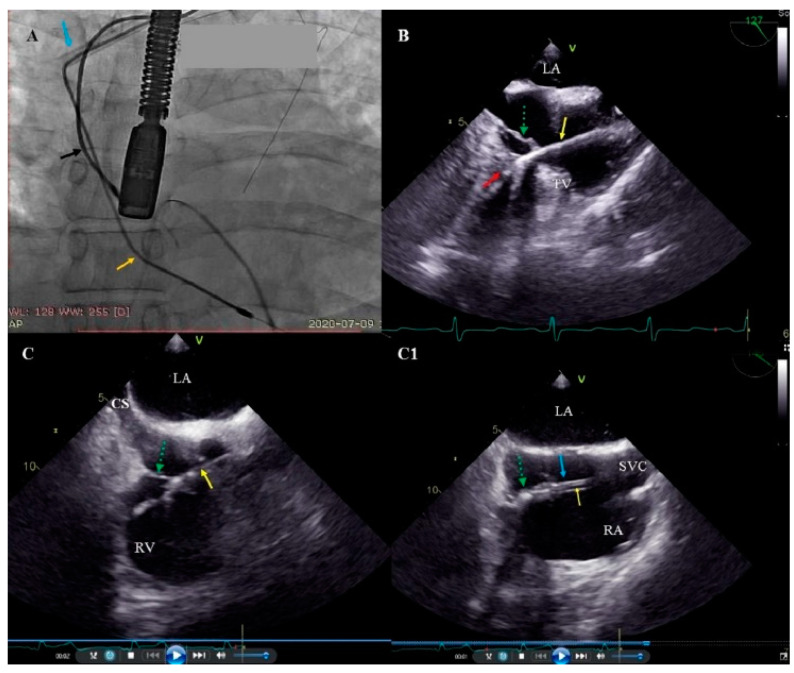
Pulling-up of the tricuspid valve (TV) leaflet while removing the lead adhered to the tricuspid apparatus. (A) Fluoroscopy. Initial phase of the removal of the ventricular lead (yellow arrow) using the Byrd dilator (blue arrow). Visibly tense ventricular lead. Atrial lead (black arrow). (B) 2D transesophageal echocardiography (TEE), mid-esophageal view. Simultaneous TEE image. Pulled-up ventricular lead (yellow arrow) with uncontrolled pulling of the septal leaflet of the TV (green arrow) lead adhesions to the septum (red arrow). (C,C1) 2D TEE, middle and low esophageal views. The next steps in removing the adherent to the posterior TV leaflet (green arrow) of the ventricular lead (yellow arrow). (C1) Winding of the leaflet (green arrow) on the dilator (blue arrow).
